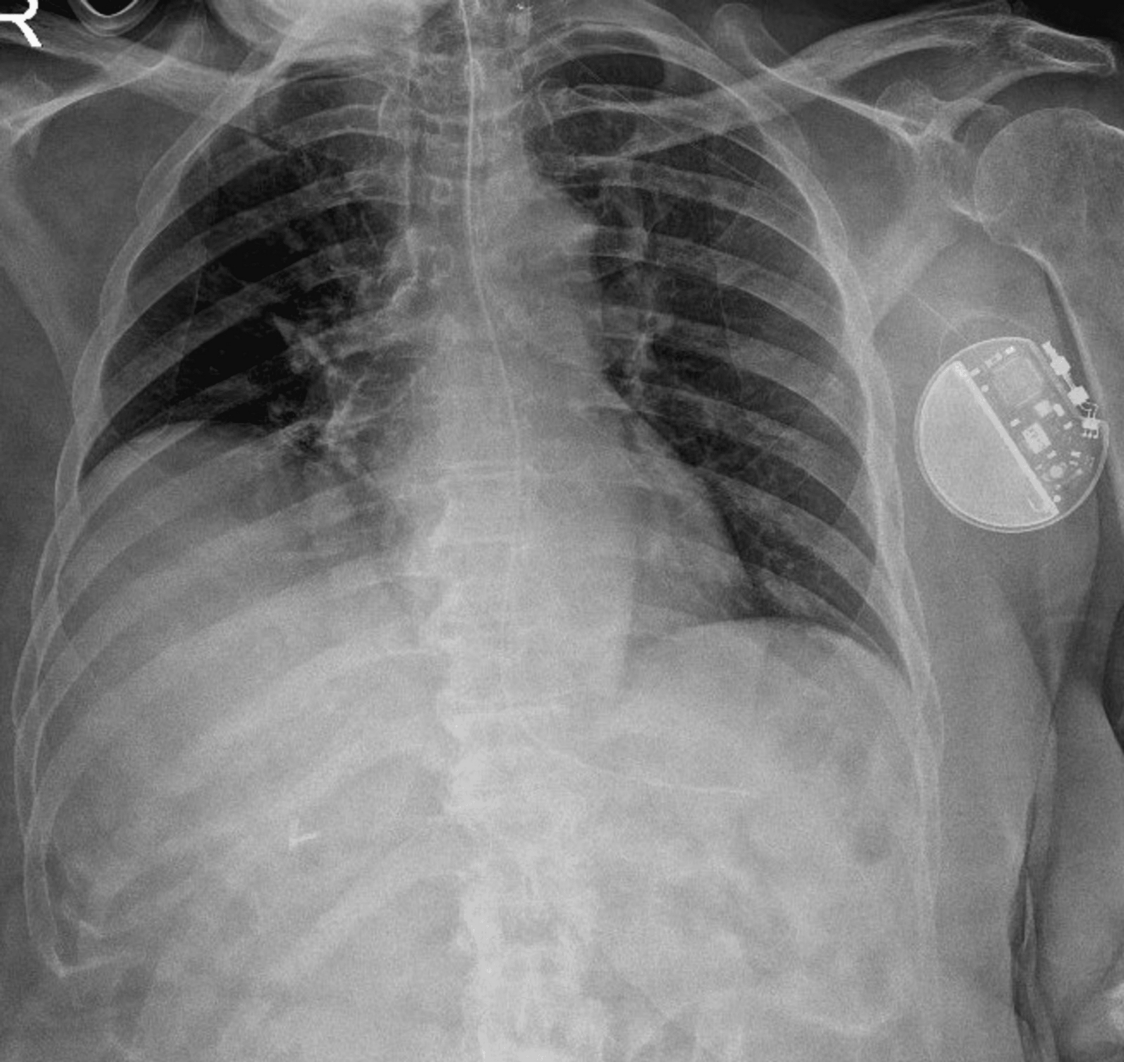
Patient chest X-ray showing the vagal nerve stimulator on the left side of the chest.
